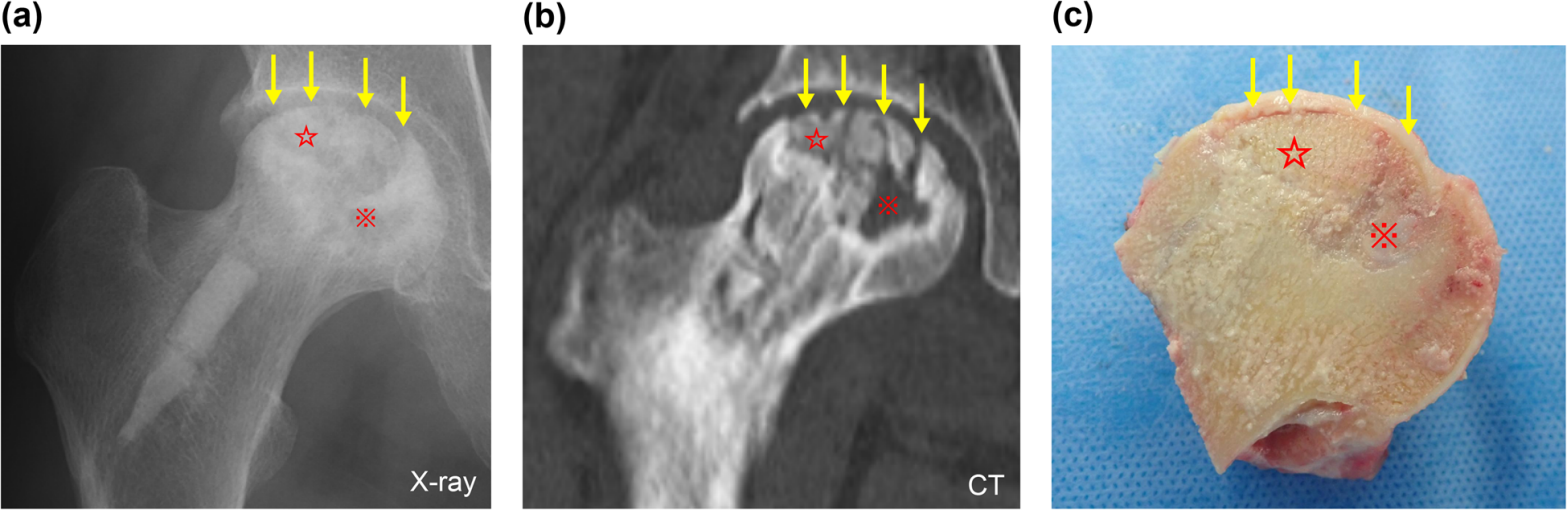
Radiographic images and a cross-sectional view of the explanted femoral head (case 20). Downward yellow arrows indicate flattening or a subchondral crack, red stars indicate the IBG site, and the red asterisk indicates the bone cyst area on the medial side of the femoral head. The graft bone remained, but the subchondral bone was flattened and detached from the cartilage. CT, computed tomography; IBG, impaction bone graft
